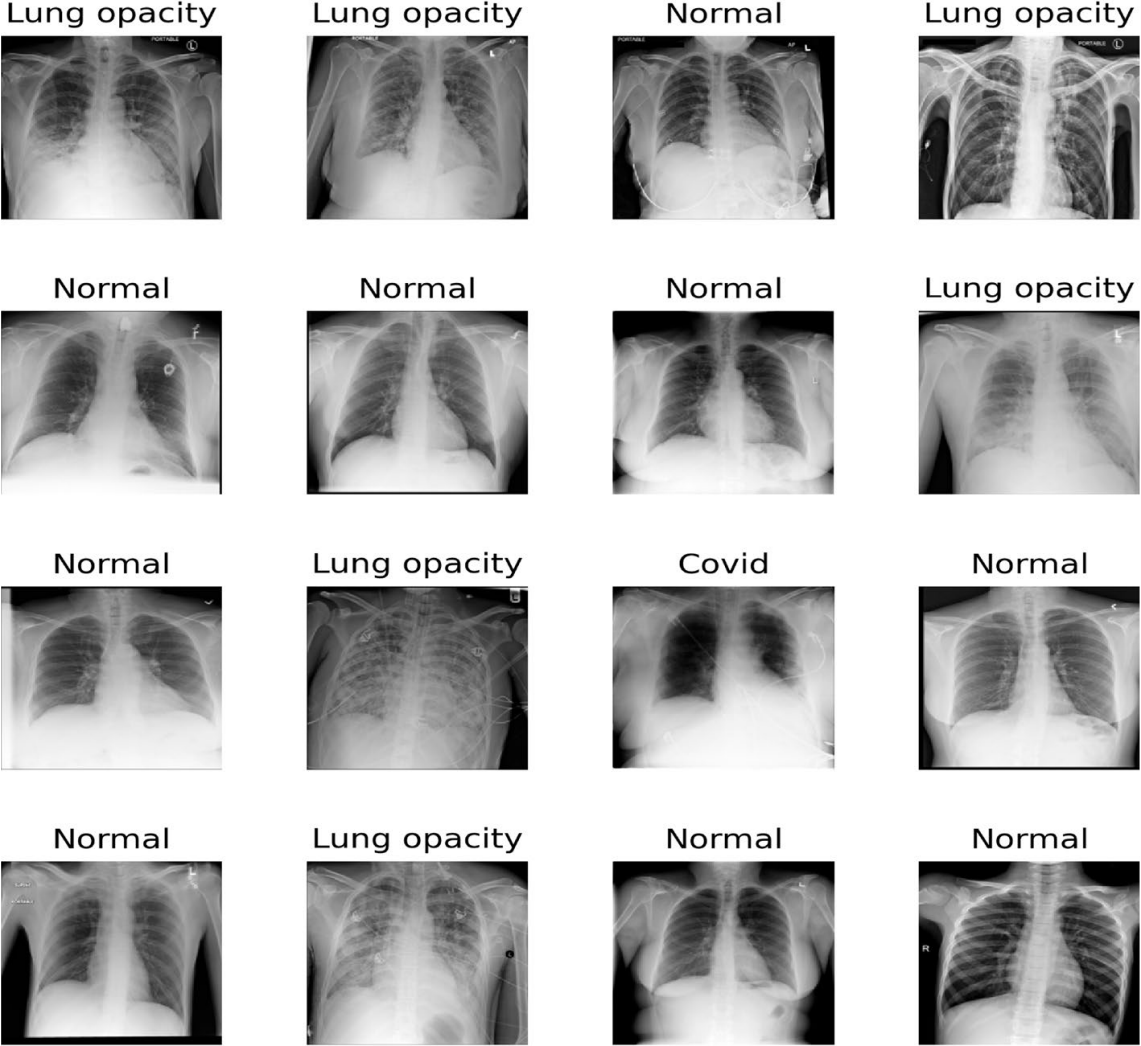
Sample chest X-ray images from the dataset used in this study, labeled with their respective conditions. The conditions include Normal, Lung opacity, and Covid. The dataset was curated from multiple international COVID-19 X-ray testing centers during several periods. The diversity in conditions showcases the varying features that the models need to identify for accurate classification
